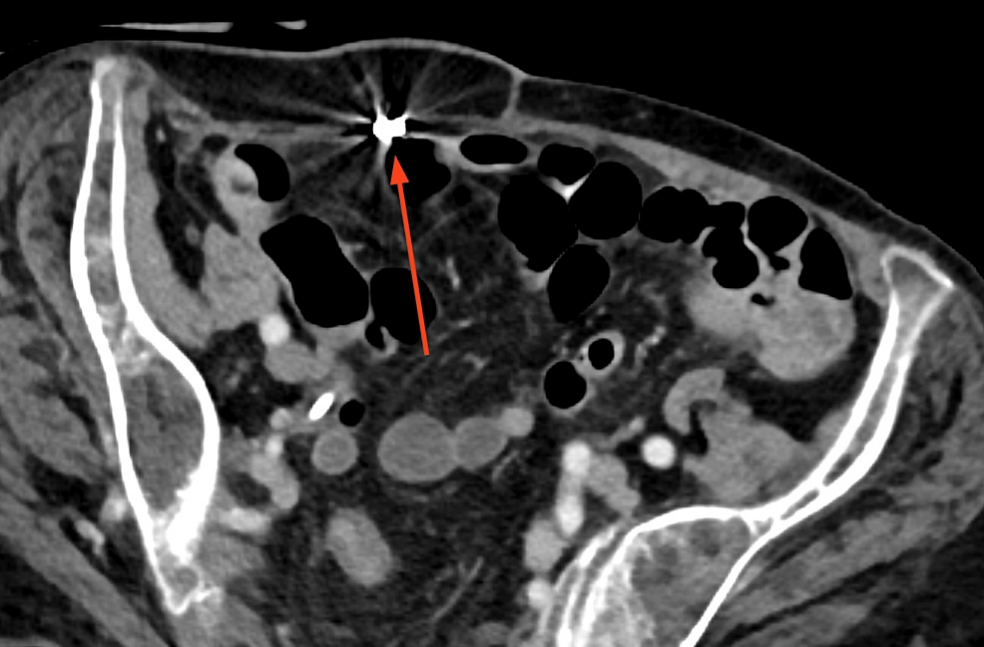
CT following surgical mesh repair of the hernia
